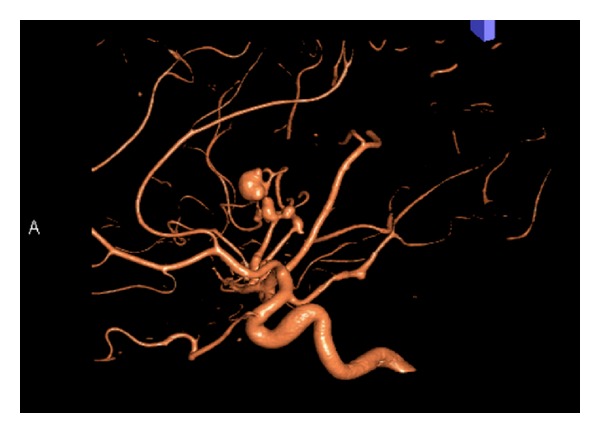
Lateral CTA scans demonstrating an ectatic fusiform dilatation of the involved MCA distal branch with aneurysm. In addition, a saccular smaller aneurysm at the origin of MCA bifurcation and another fusiform smaller aneurysm at the first segment of PCA were demonstrated.
